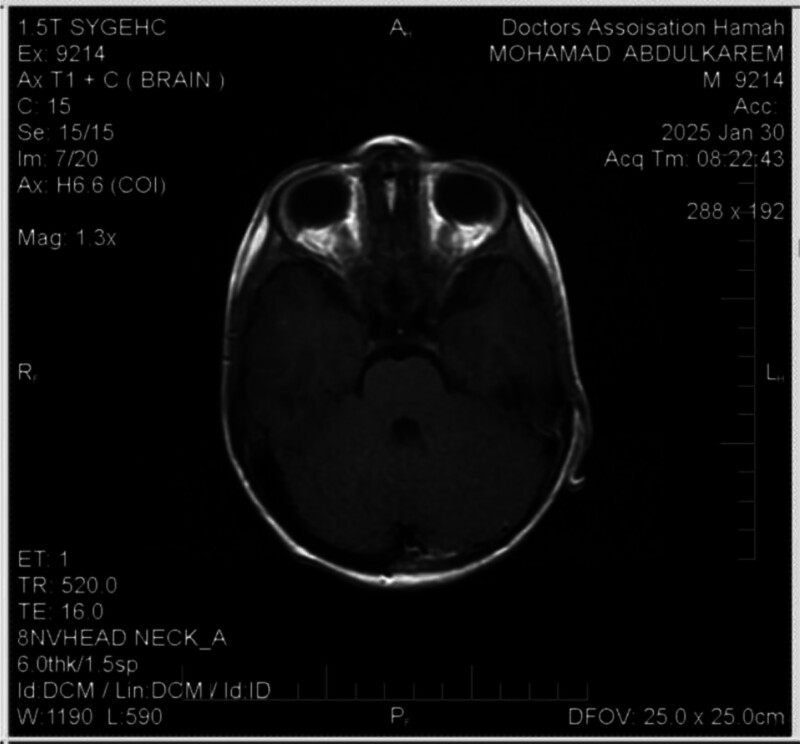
Is a follow-up MRI that shows resolution of intracranial metastasis. MRI = magnetic resonance imaging.
